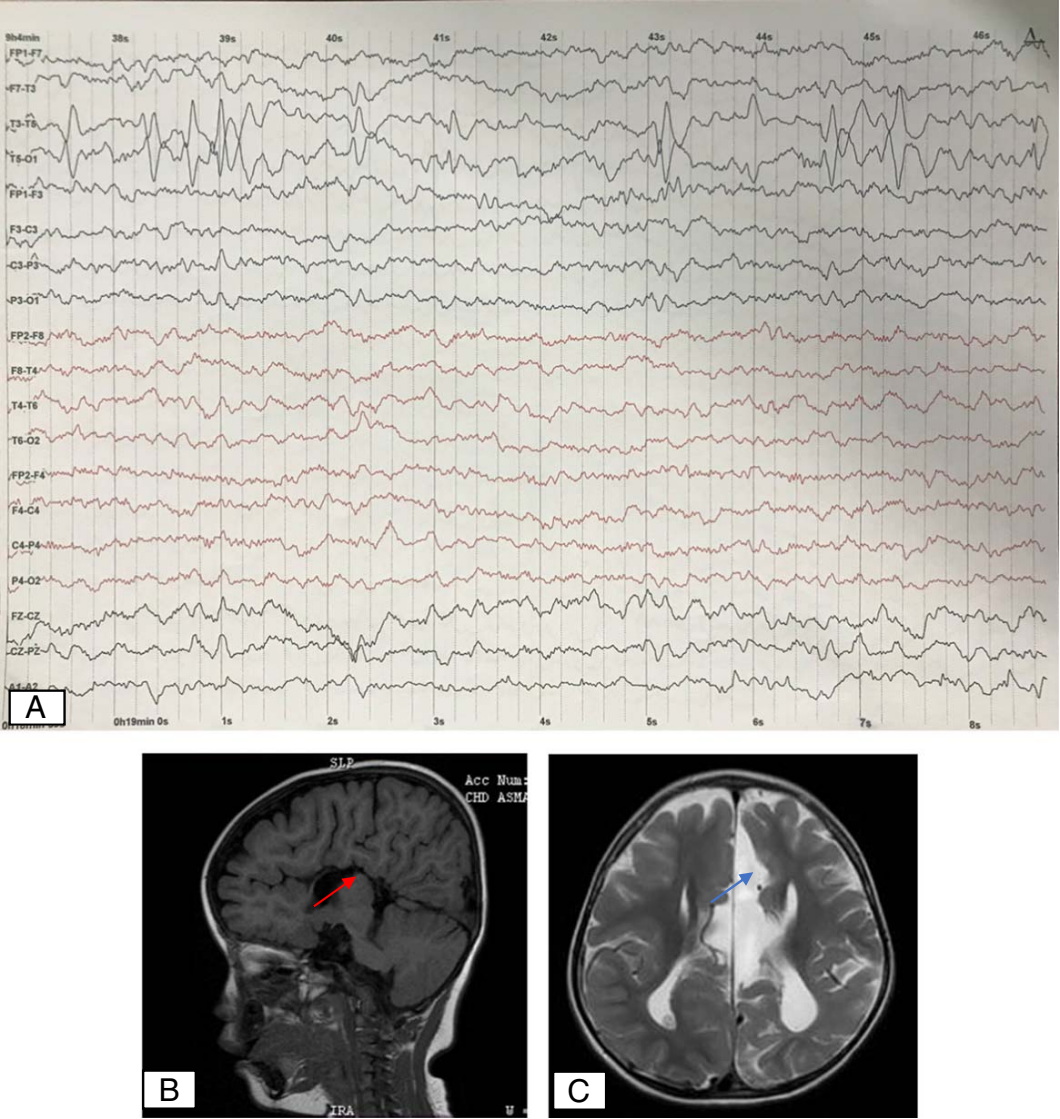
(A) Electroencephalography showed generalized epileptiform discharge on left temporo-occipital. (B) Sagittal T1-weighted image sequence. (C) Axial T2-weighted image sequence brain MRI showed corpus callosum hypoplasia (red arrow), left parafalcine cyst (blue arrow), and cerebral atrophy.
